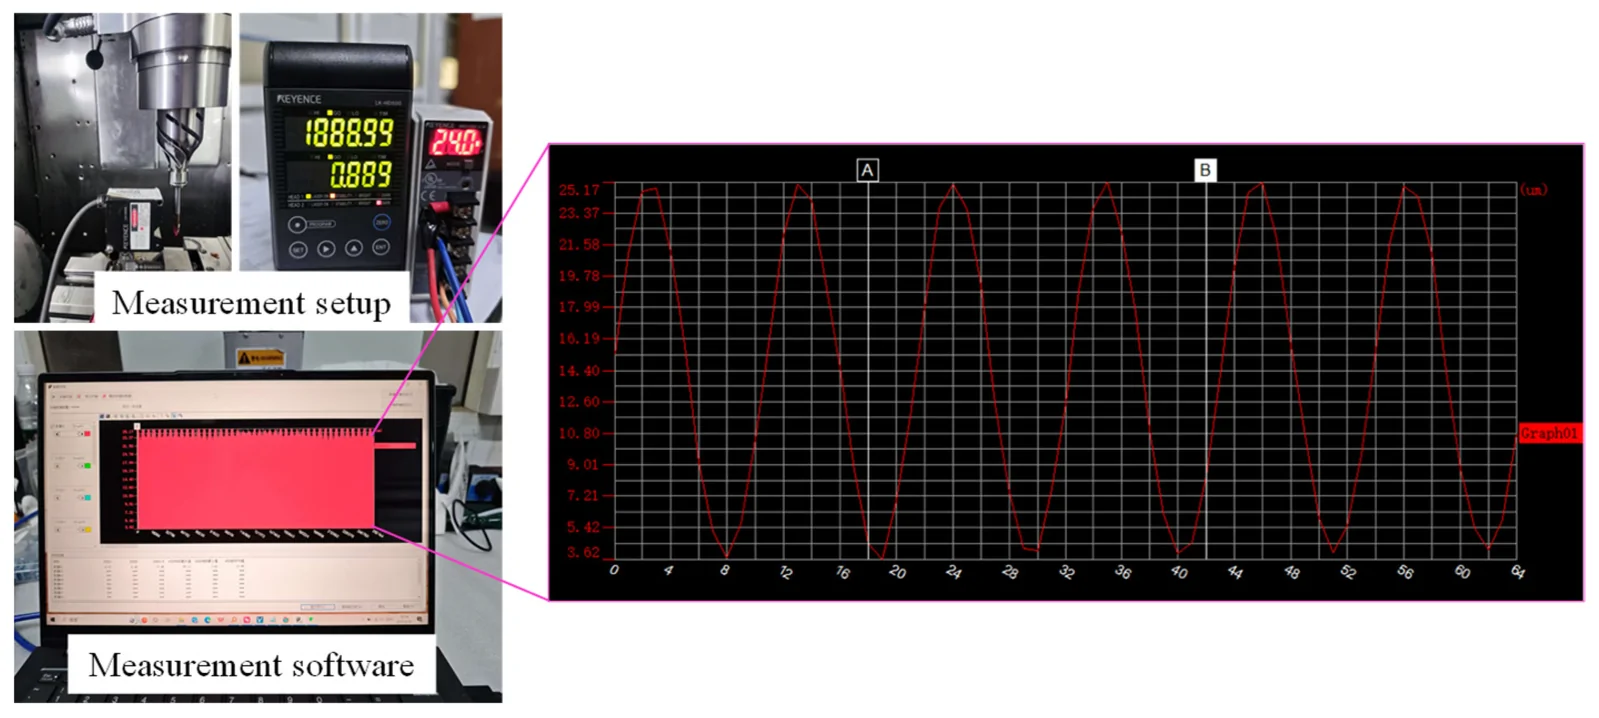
The measurement setup of longitudinal torsional ultrasonic parameters.
